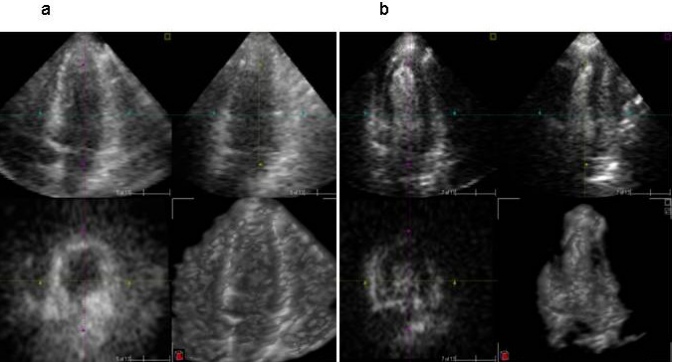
Realtime 3D echocardiography without (a) – [additional file 6], and with contrast enhancement (b) [additional file 7]. There is clear benefit for LV border detection. [See also additional files 8 and 9 for real time 3-D movies without and with contrast respectively.]
